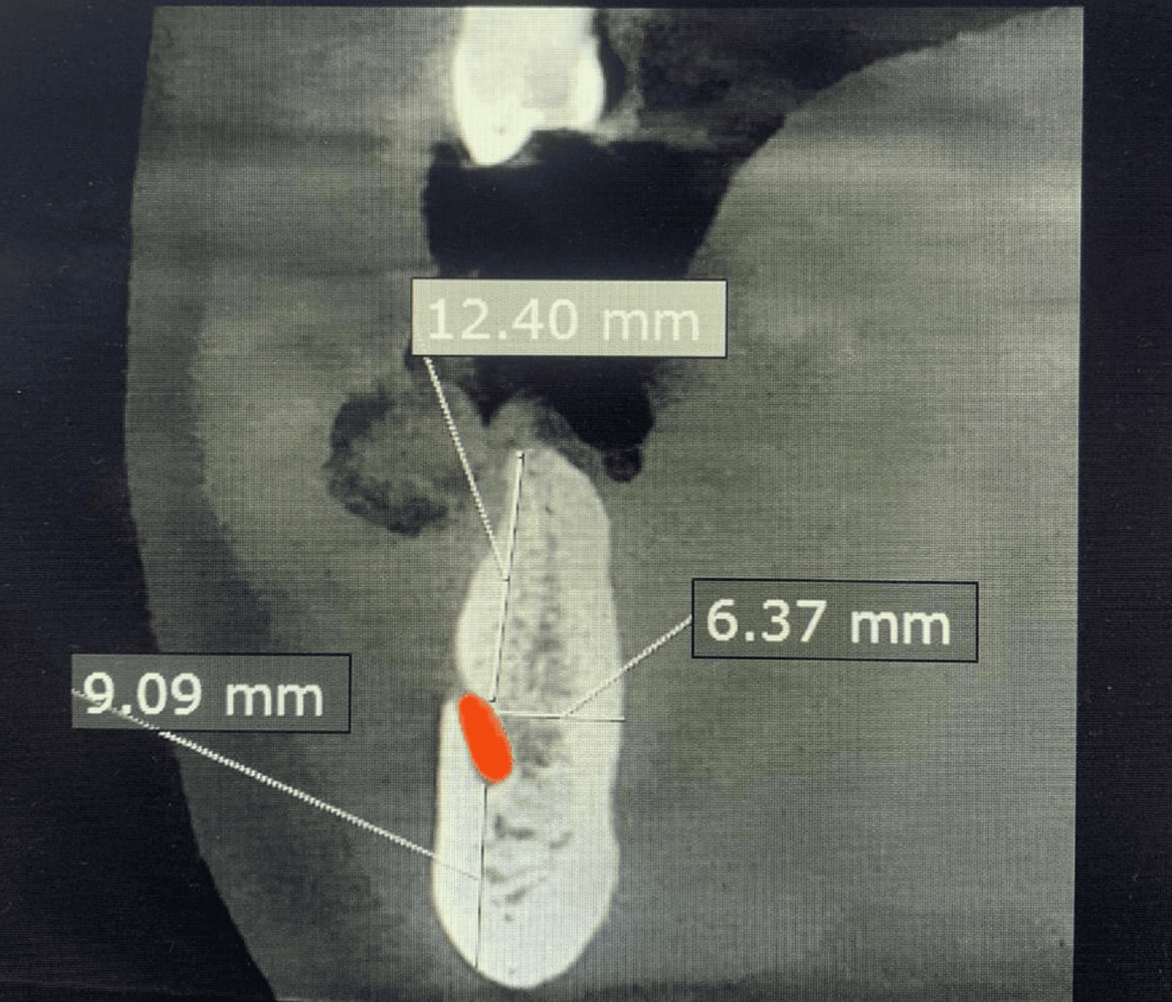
A sagittal cut from the original CBCT image showing the linear measurements of the various dimensions of the mandible surrounding the mental foramen. Mental vertico occlusal (12.4 mm), mental vertico apical (9.09 mm), and mental horizontal (6.37 mm). CBCT: cone-beam computed tomography; IAC: inferior alveolar canal
